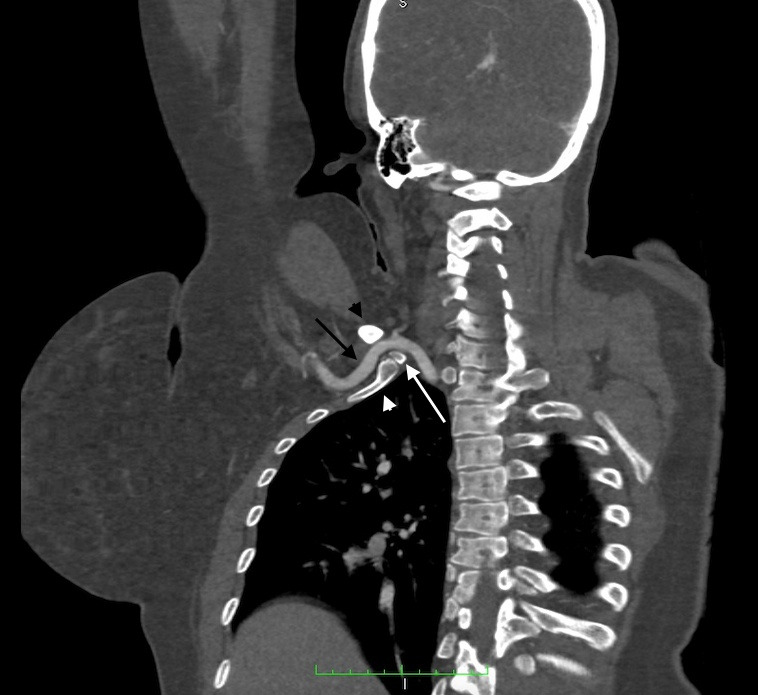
Computed tomography angiography of the thoracic region with upper limb hyperabduction in the first case. The coronal view shows compression of the right subclavian artery (black arrow), by the clavicle (black arrowhead), first rib (white arrowhead), and cervical rib (white arrow). Mild thickening of the artery wall was also observed, but without causing significant stenosis. No aneurysm was observed.
